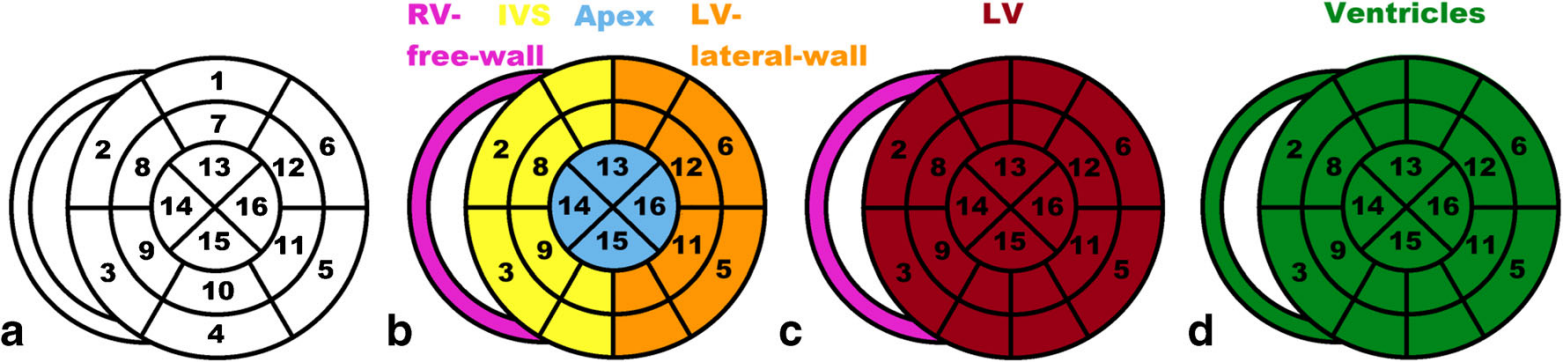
Different regions are presented in the following colours: purple—right ventricular free wall (RV free wall); yellow—interventricular septum (IVS); blue—apex of the heart (Apex); orange—left ventricular lateral wall (LV lateral wall). The definition of these follows stepwise. A. Description of the echocardiographic 16-segment short axis bulls eye model. B. Regions used in the study. The anterior segments [1, 7] and the posterior segments [4, 15] were split in half and joined with their respective side. C. Regions used to compare uptake against echocardiographic strain: red—left ventricle (LV), purple—right ventricular free wall (RV free wall). D. Region used to compare uptake against cardiac biomarkers: green—heart ventricles (whole heart)
